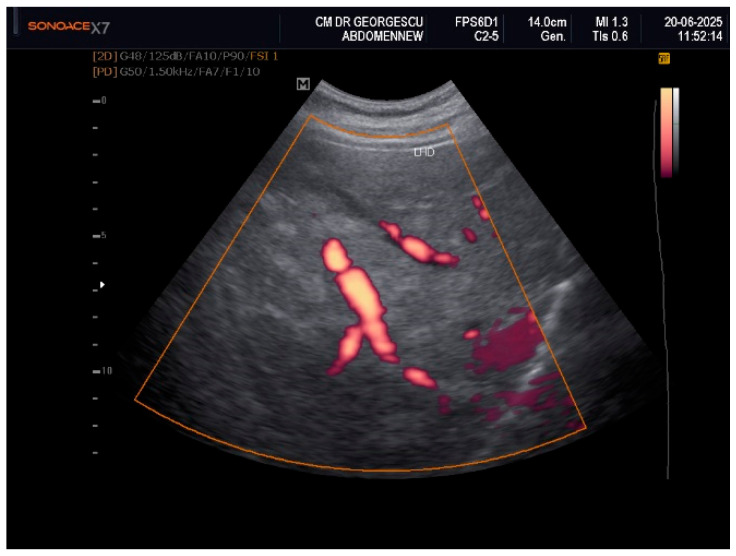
Power Doppler: No mass effect or invasiveness of liver hyperechoic nodules.
